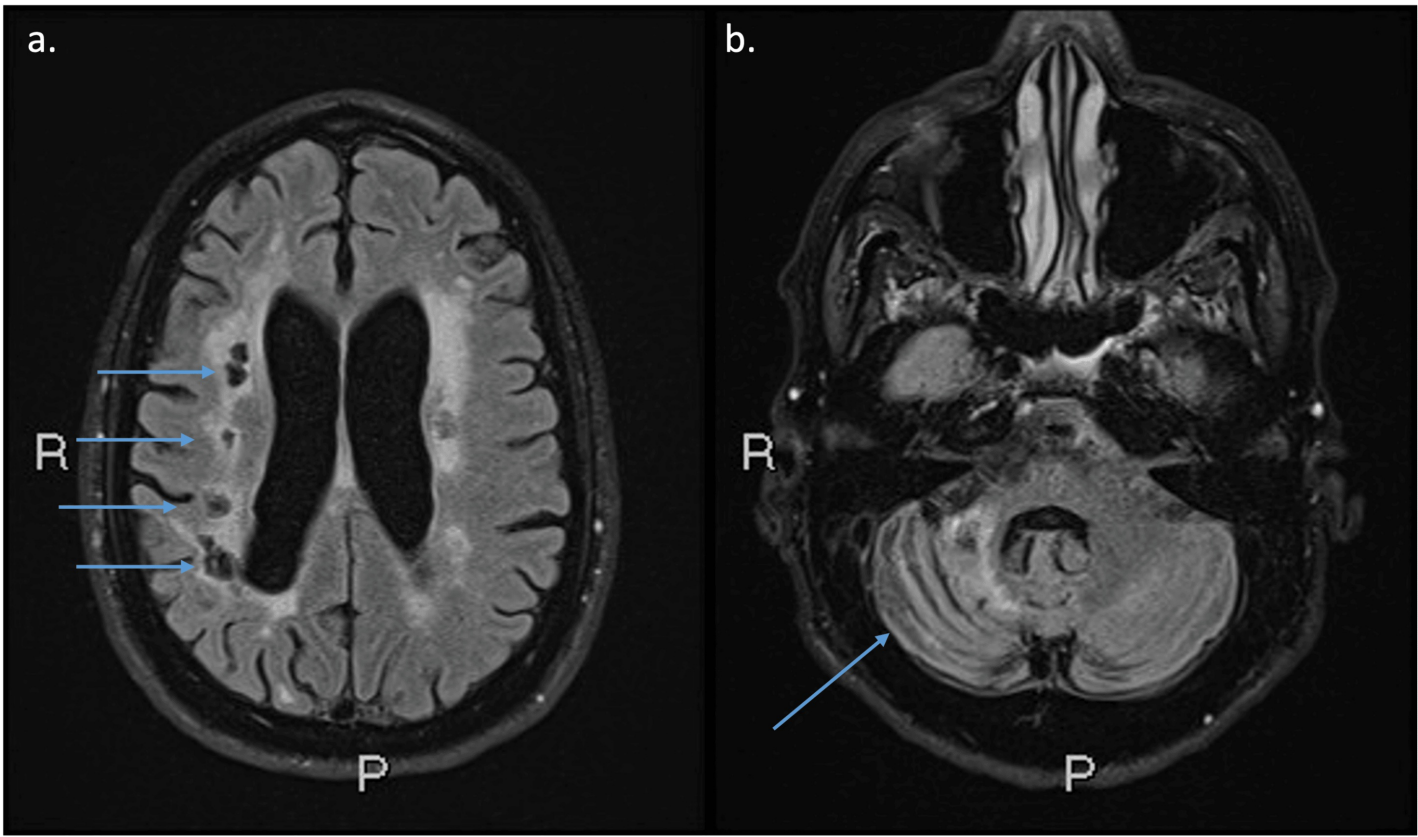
Axial T2 FLAIR fat-saturated MRI images. Axial T2 fluid attenuated inversion recovery (FLAIR) fat-saturated MRI images demonstrate multiple chronic infarcts (arrows) in the right coronal radiata (a) and a chronic infarct in the right cerebellum (b). The patient also had a focus of infarct in the left corona radiata and right thalamus (not pictured). The patient demonstrated mixed transcortical aphasia, which can likely be explained by involvement of the left arcuate fasciculus.
